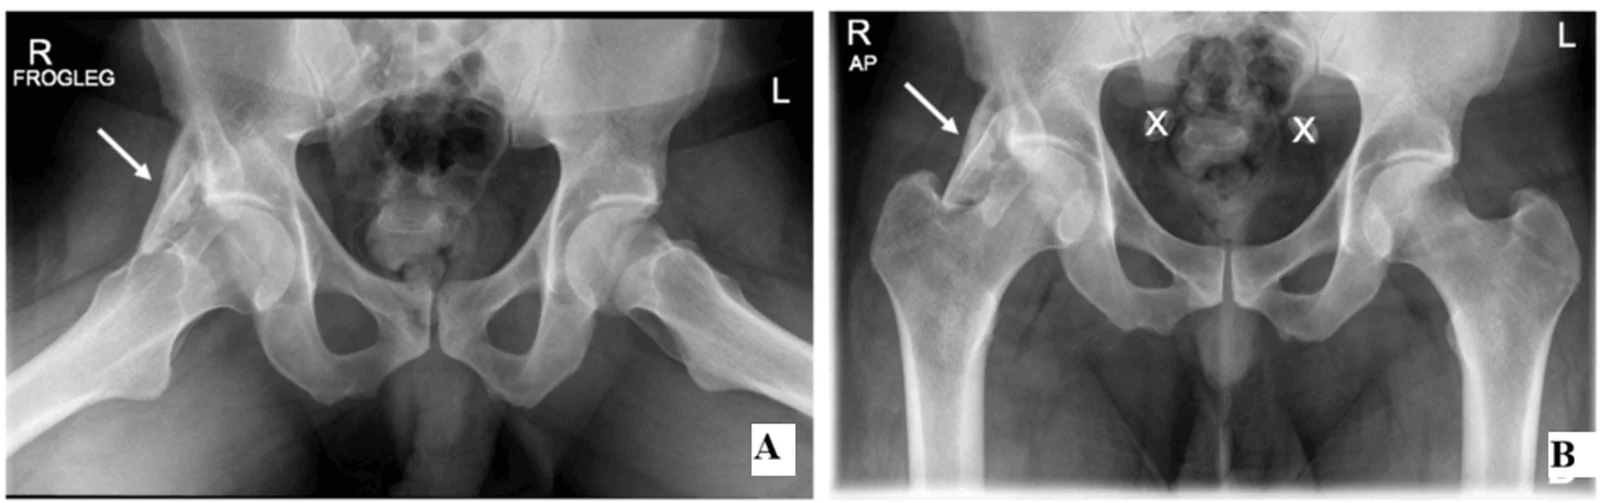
Plain radiographs of the pelvis, showing a well-defined bony bar anterosuperior to the right hip joint with pseudoarthrosis with the right anterior inferior iliac spine (pathognomonic of pelvic digit). (A) Frog view. (B) AP view AP: anteroposterior
